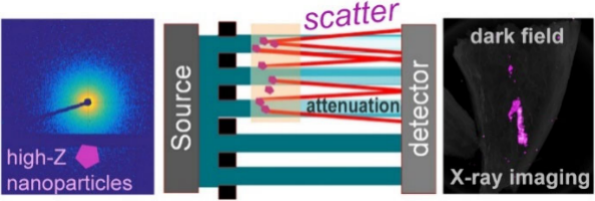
The poor soft tissue contrast of X-ray CT necessitates
